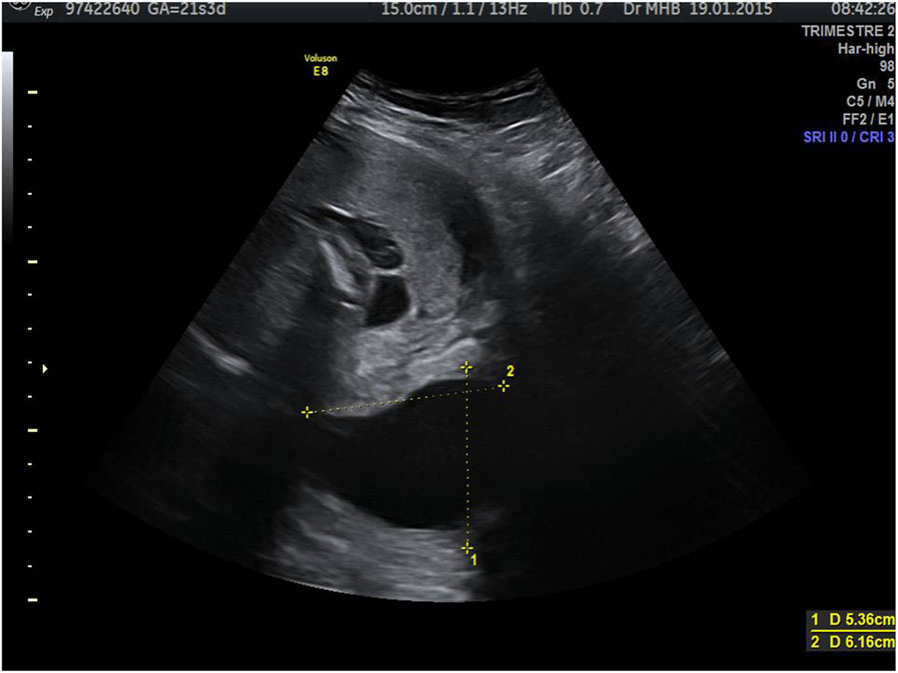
Cervical ultrasound at 21 3/7 weeks showing a long cervix with bulging membranes through the pessary
